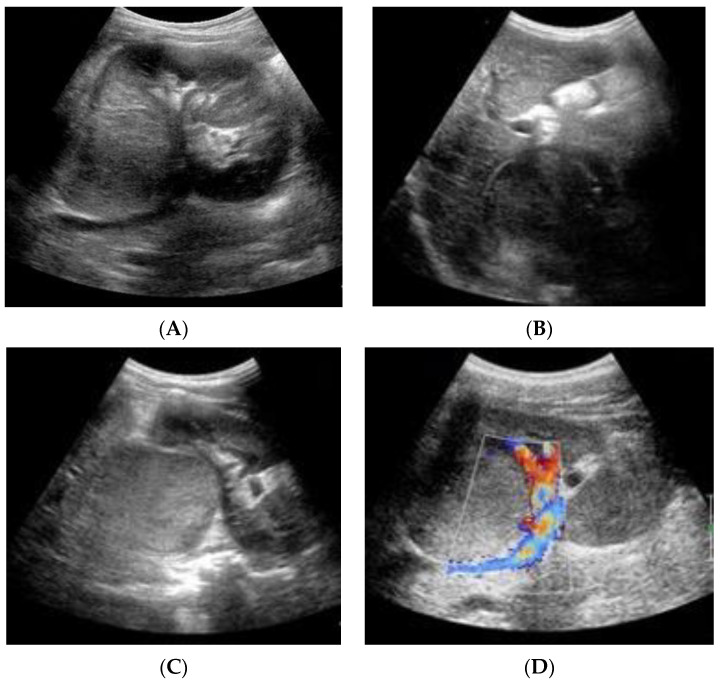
(A–D). Abdominal US: (A) A solid, roundish mass is visible at the upper pole of the right kidney. (B) The mass exhibits homogeneous isoechoic content, with an easily distinguishable pseudocapsule. No signs of involvement with neighboring organs are recognized. (C) Transverse scan of the tumor. (D) The color Doppler shows no evidence of renal vein thrombosis.
